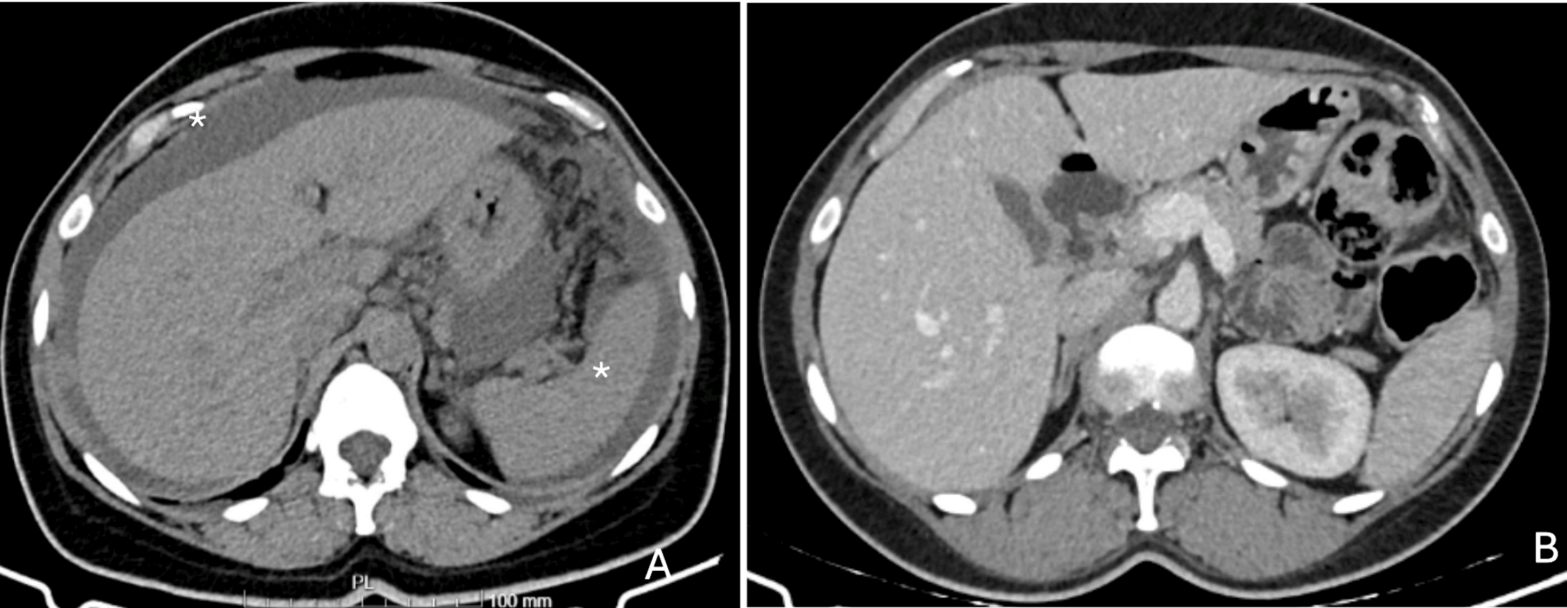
CT-TAP at diagnosis (A) and after complete cytoreductive surgery (B) (A) Axial contrast-enhanced thoracoabdominal CT at diagnosis demonstrating large-volume ascites (*), and extensive peritoneal involvement, with free fluid surrounding the liver and spleen. (B) Axial postoperative CT-TAP following complete cytoreductive surgery, showing resolution of ascites and no radiological evidence of residual peritoneal disease, consistent with a complete radiologic response. CT-TAP: computed tomography of the thorax, abdomen, and pelvis
